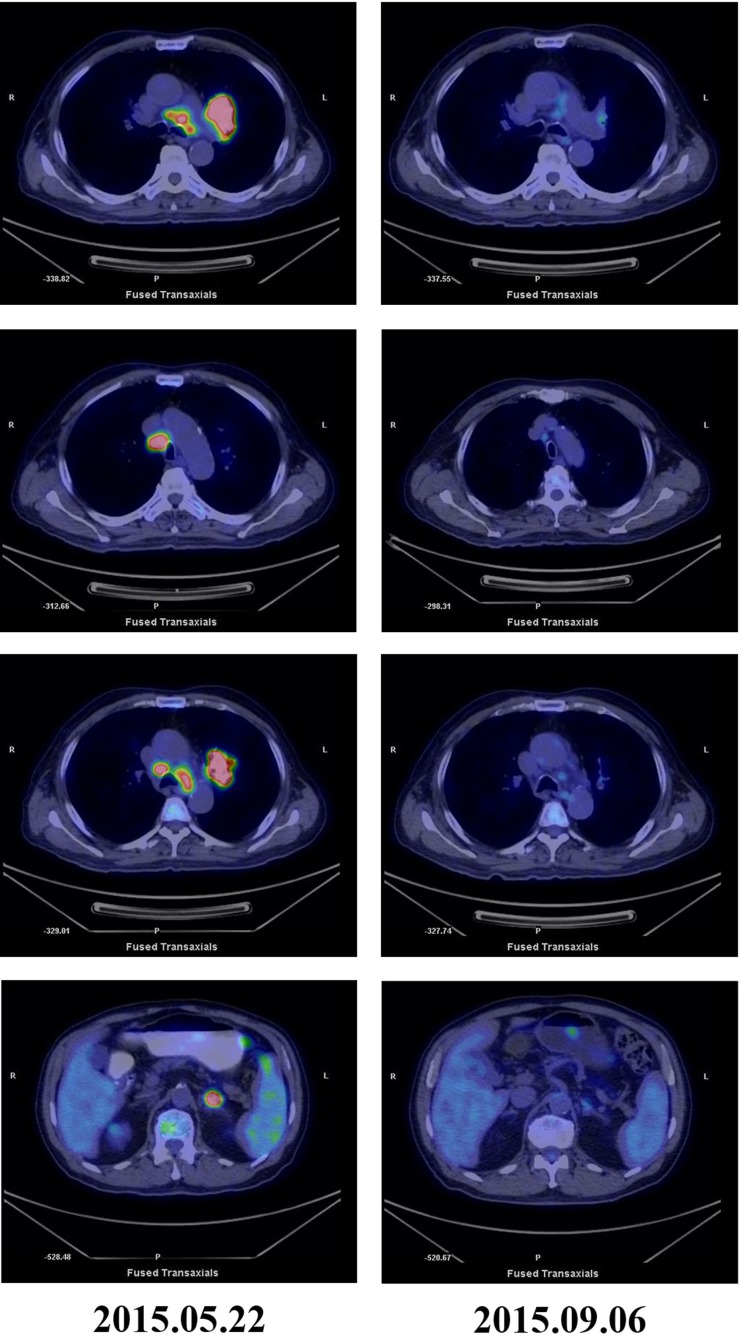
PET-CT scans before and after treatment. PET-CT May 2015 before treatment showing high glucose metabolism in left hilar mass, multiple mediastinal lymph nodes, and left adrenal masses. PET-CT September 2015 after five courses of pembrolizumab and three courses of CIK cell therapy showing significantly reduction in tumor mass and FDG uptake.
